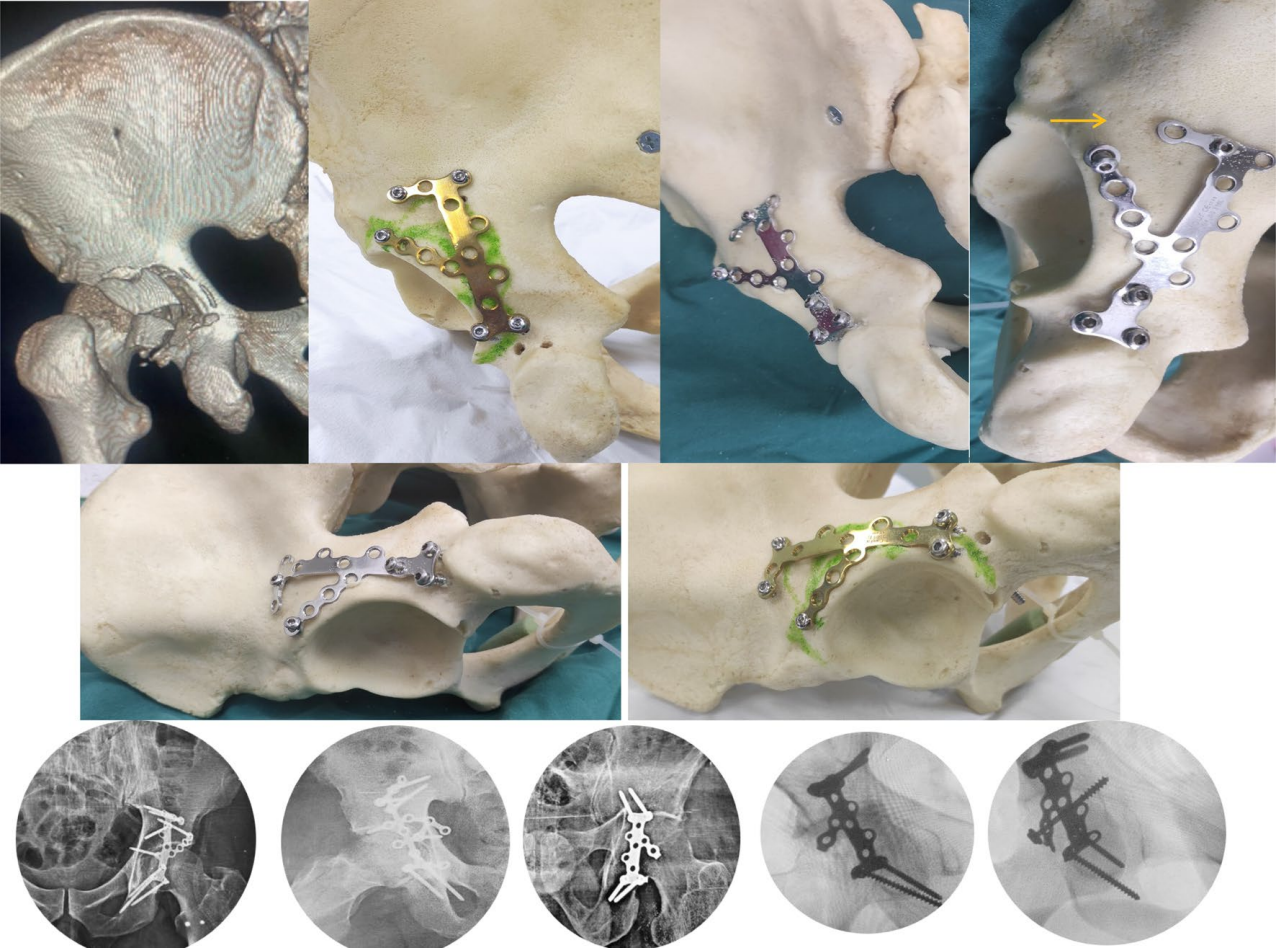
Saw bone demonstration showing calcaneal plate application with large footprint area to cover all expected posterior wall fragments and case examples radiograph
